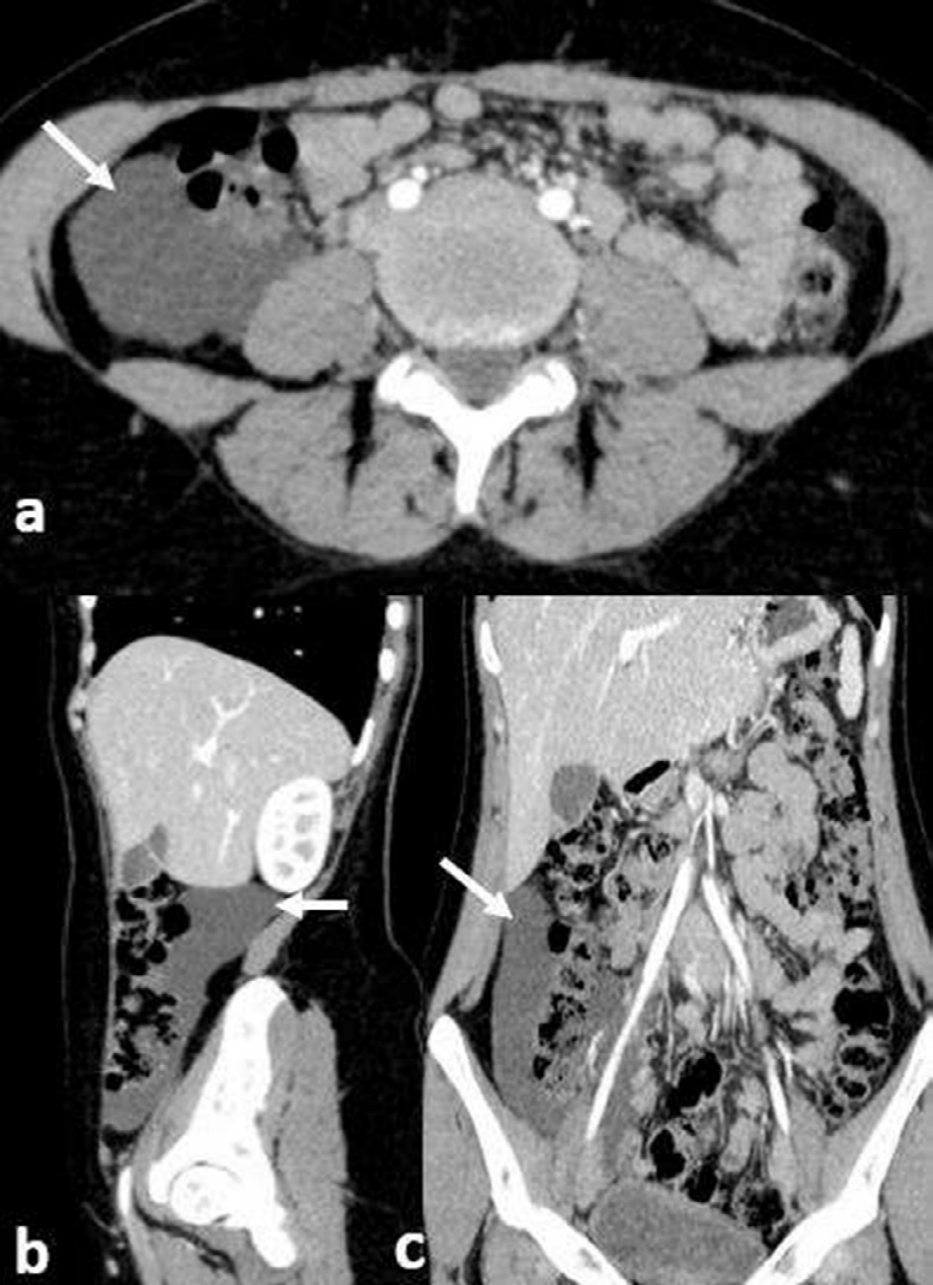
(a, b, c) Abdominal CT scan with i.v. contrast on axial plan, with sagittal and coronal reconstructions showing a multilocular cystic mass (white arrows) with a low-density and fluid density (4 HU), without enhancement neither visible wall, located at the ascending colon. HU, Hounsfield unit.
